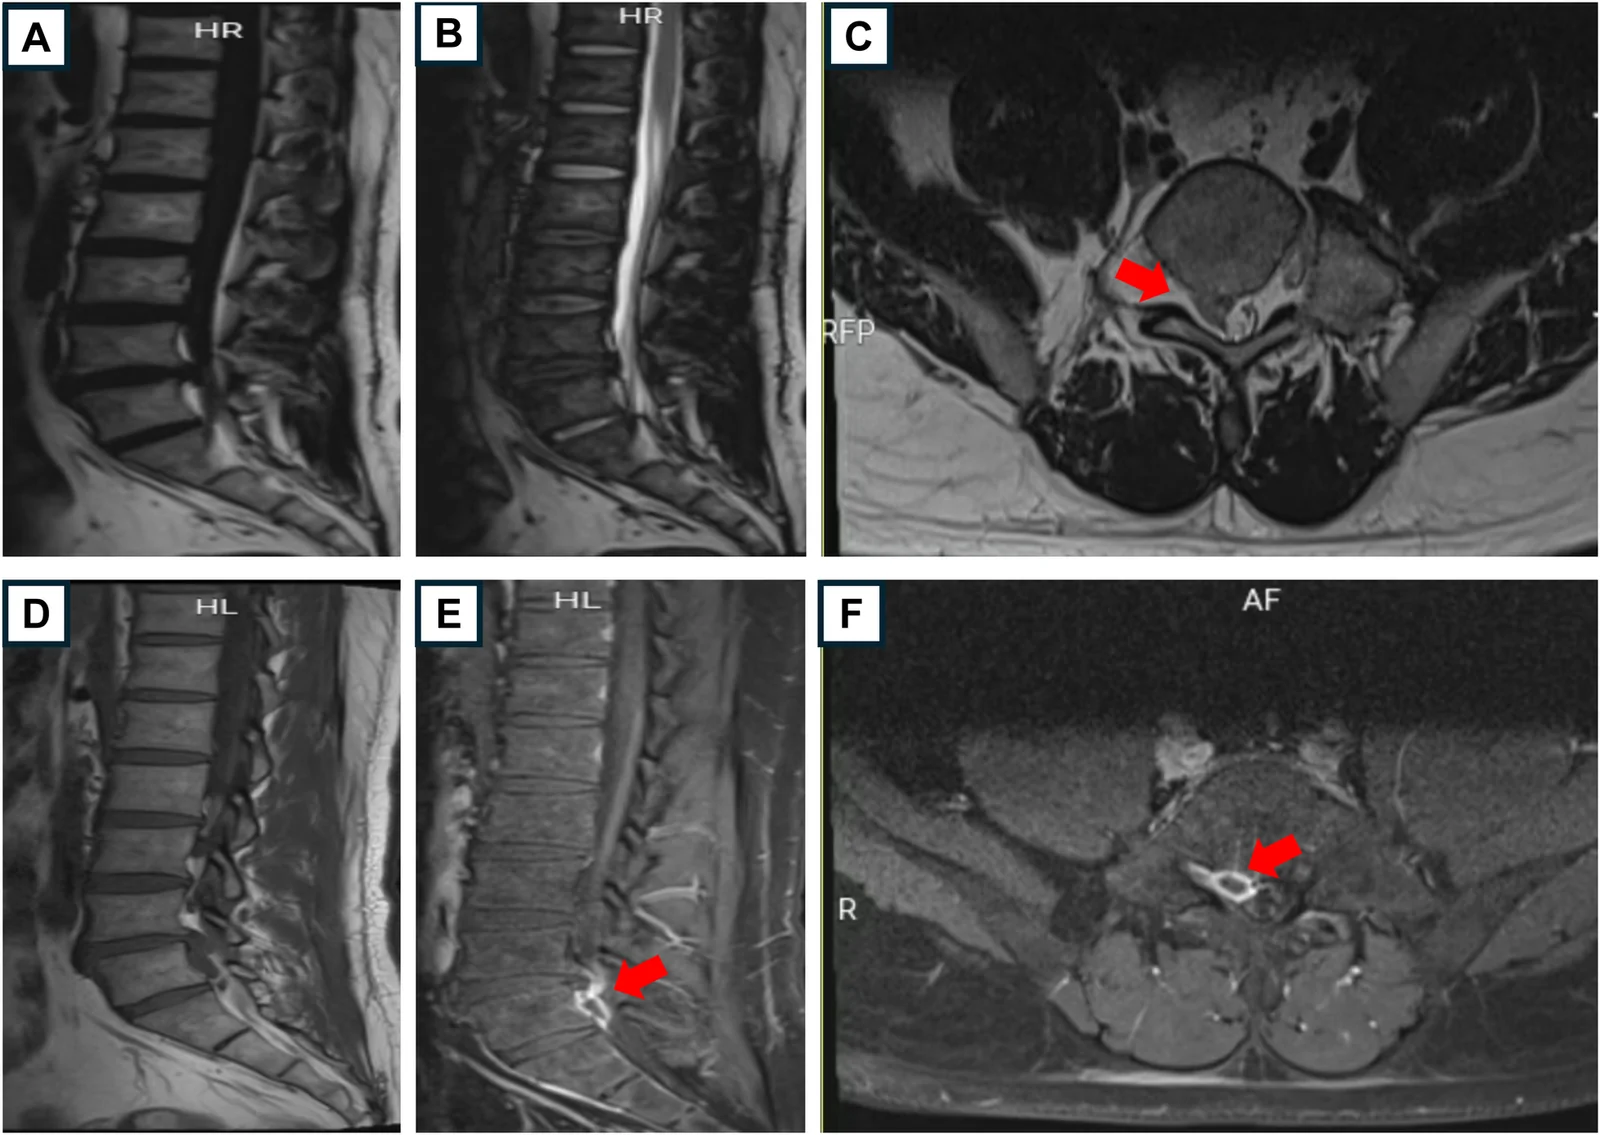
Initial lumbar MRI findings. Non-contrast MRI (June 16, 2025) and contrast-enhanced MRI (June 19, 2025) demonstrate an L4/5 disc extrusion with sequestration. (A–C) Axial non-contrast images show a herniated nucleus pulposus occupying 40.6% of the spinal canal (red arrow). (D,E) Sagittal contrast-enhanced images indicate partial sequestration at the L4/5 level (red arrow). (F) Axial contrast-enhanced image reveals peripheral rim enhancement surrounding the sequestrated disc fragment, a classic radiographic phenomenon referred to as the “bull's-eye sign” (red arrow).
